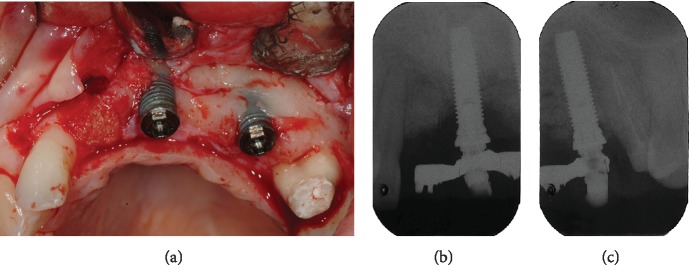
The regenerated bone after 14 years of loading, during surgery to treat the peri-implantitis lesions on the buccal surface of the implants and after extraction of the right central incisor: (a) clinical view, (b) periapical radiograph of mesial implant, and (c) periapical radiograph of distal implant.
